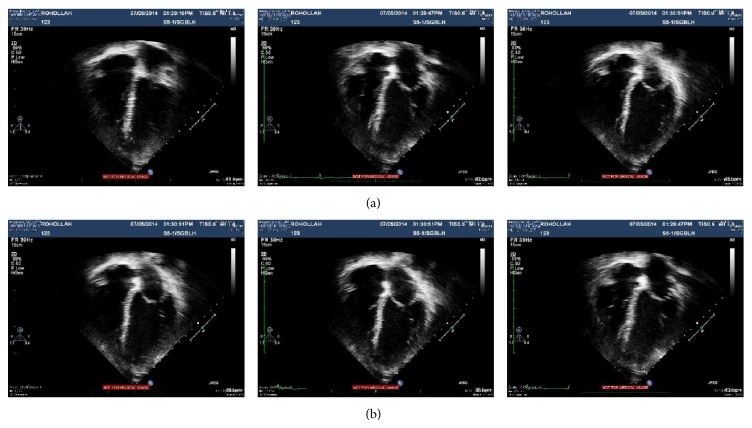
Original cardiac ultrasound images; (a) first row, dataset number 1, and (b) second row, dataset number 2.
